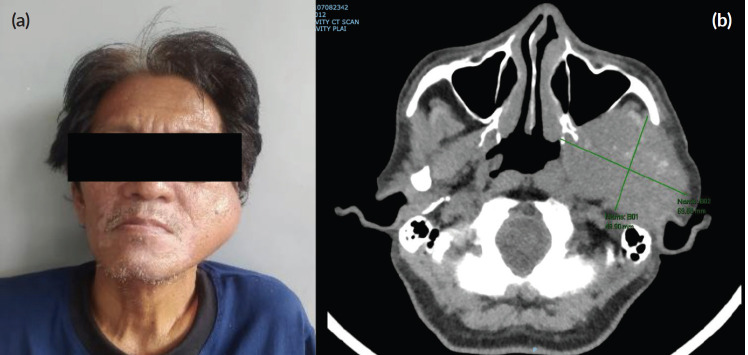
(a): Rapidly growing left facial mass associated with occasional pain and trismus. (b): CT scan of the left parotid mass with osseous destruction of the mandible.
